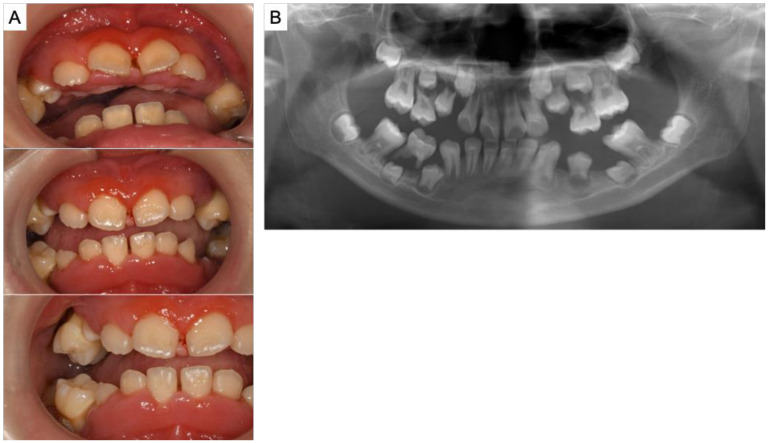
Initial examination at the age of 8 years and 5 months. (A) Intraoral photographs. (B) Panoramic radiograph.
